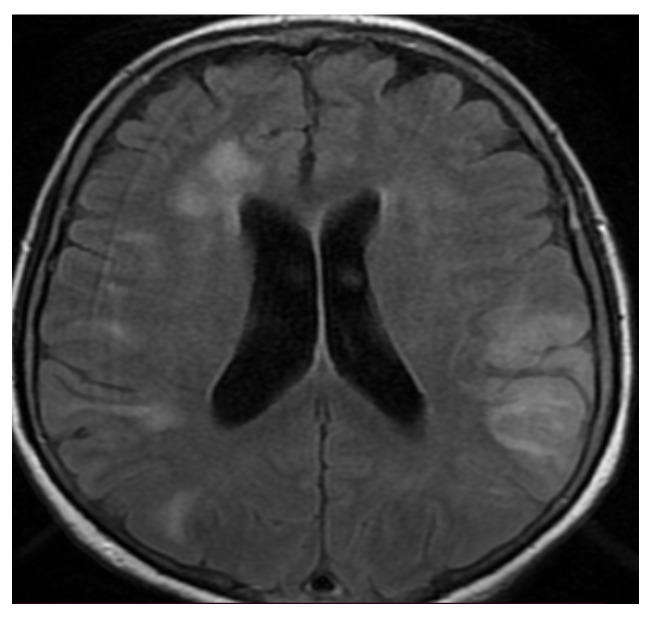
MRI axial fluid-attenuated inversion recovery (FLAIR) image showing bilateral subcortical hyperintense lesions in the frontal, parietal, and temporal lobes, without significant enhancement, compatible with encephalitis. MR venography 2D-TOF (2D time-of-flight) excluded cerebral venous sinus thrombosis.
